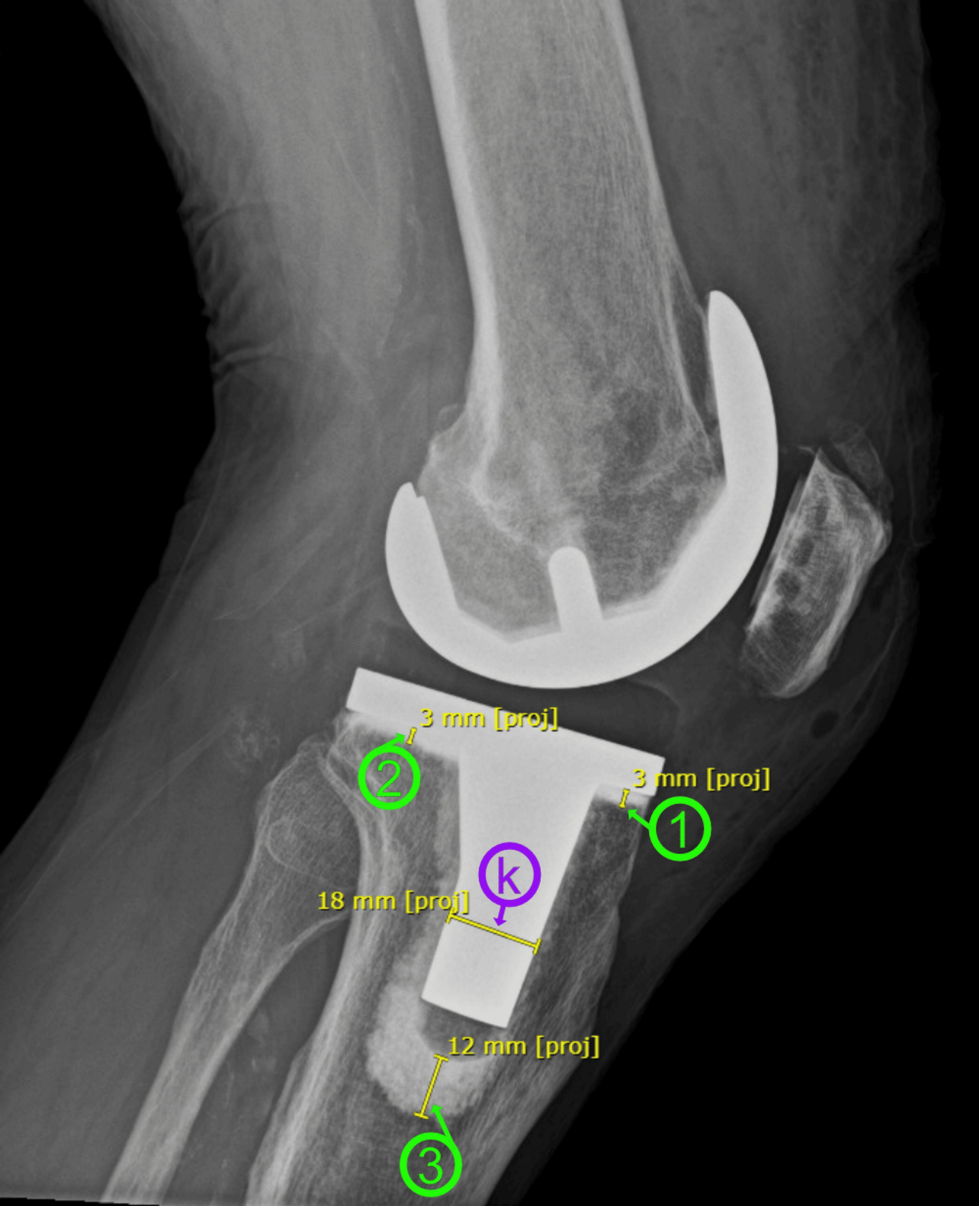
Measurement of cement penetrations on lateral knee X-rays The green circles containing numbers 1-3 indicate the measured respective zones from zone 1 to zone 3. The purple circle containing the letter "k" indicates the keel diameter measurement. Based on the Knee Society Roentgenographic Evaluation System [16].
